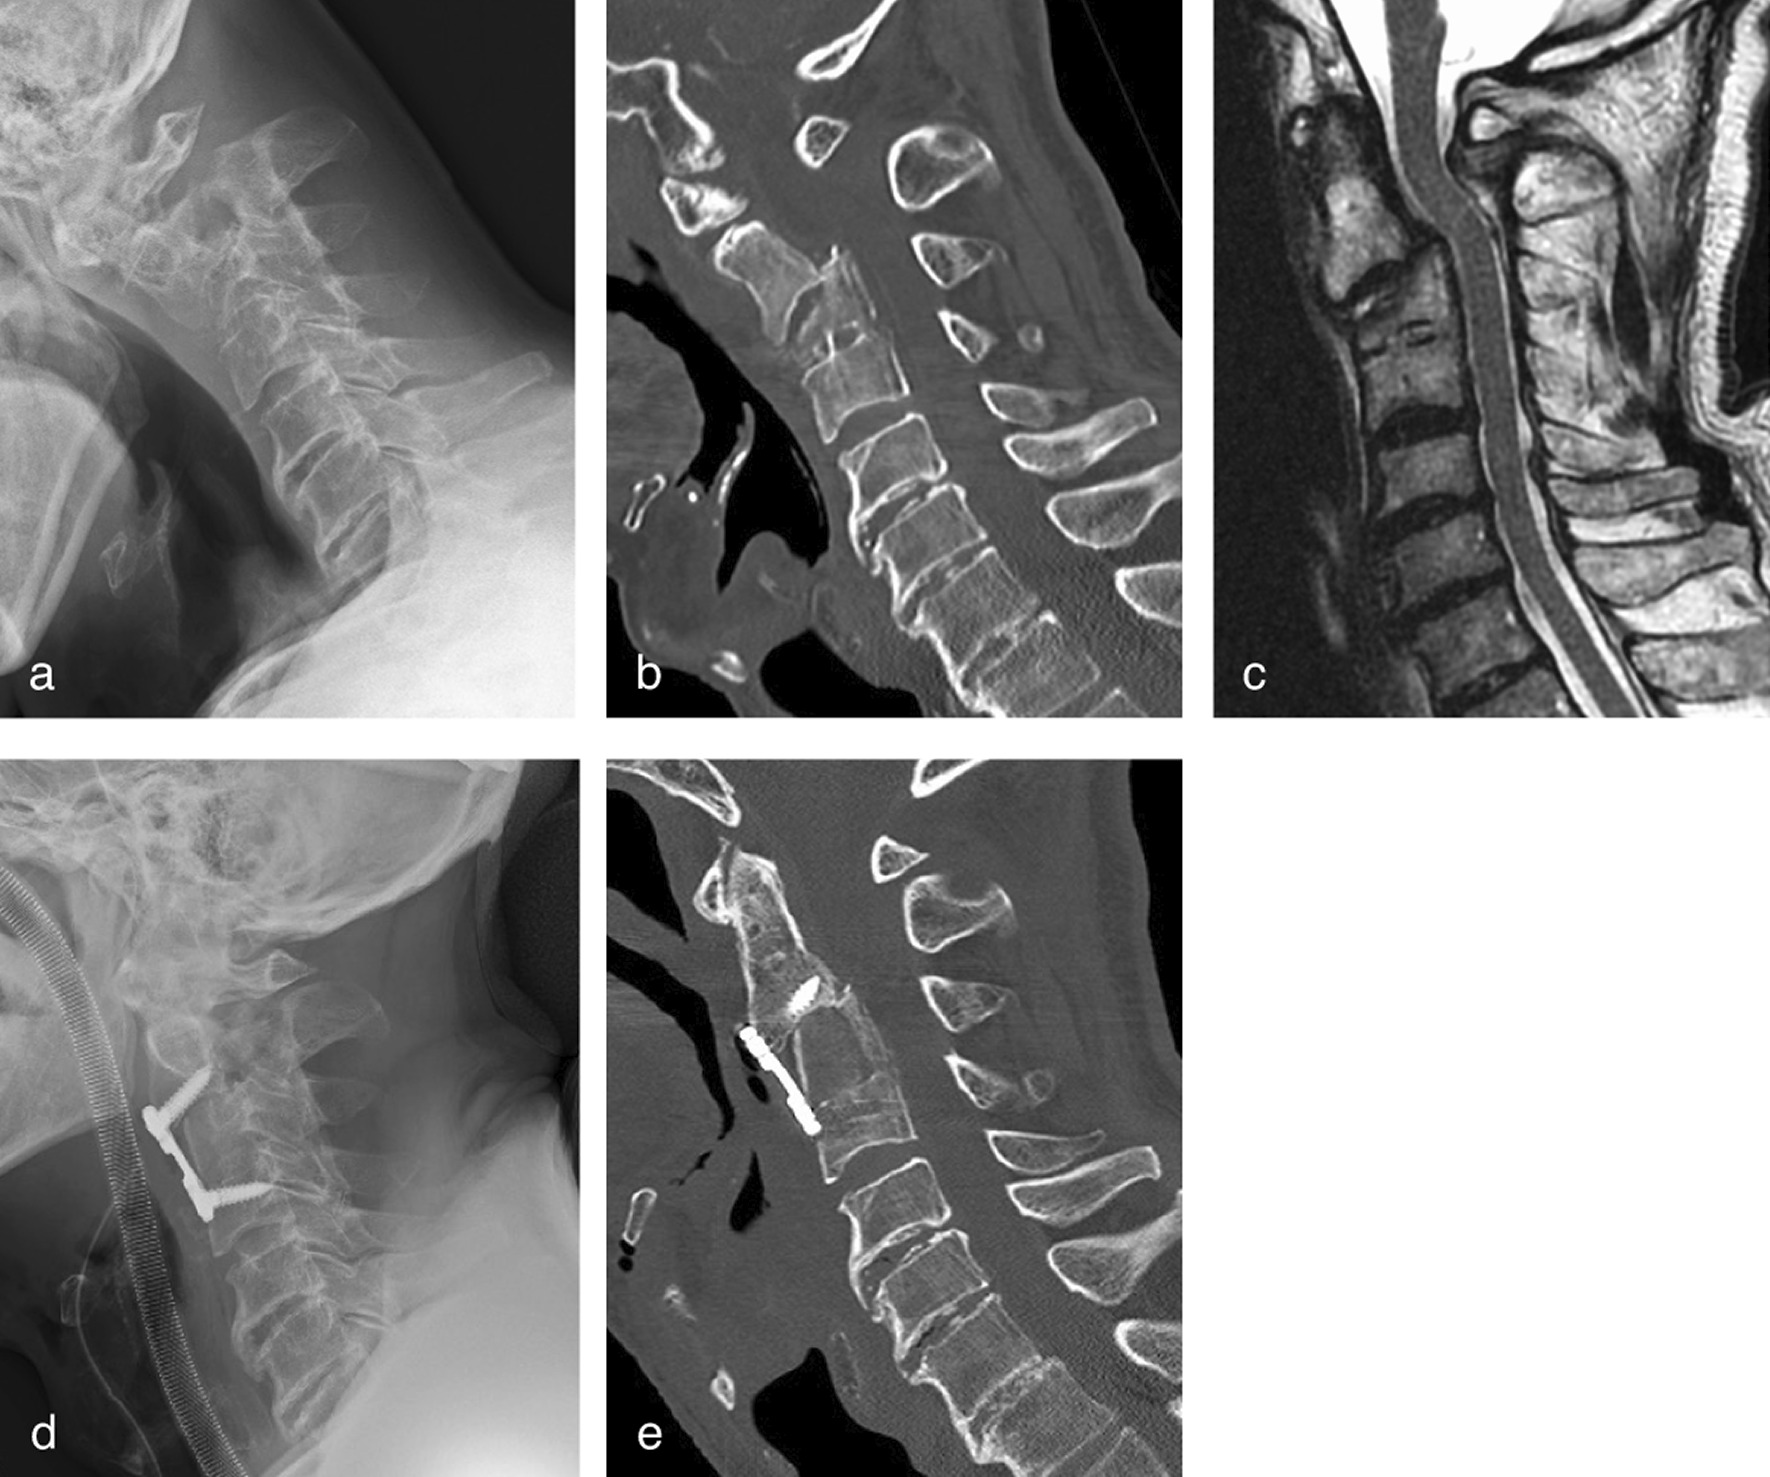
Case 10. a Preoperative lateral plain radiographs and b computed tomography sagittal images showing severe kyphotic deformity with C2 anterolisthesis and C3 vertebral collapse. c T2-weighed magnetic resonance sagittal image showing sigmoid course of the spinal cord without compressive lesion. d Postoperative lateral plain radiograph and e computed tomography sagittal image showing precise iliac bone grafting and C2–4 plate fixation
